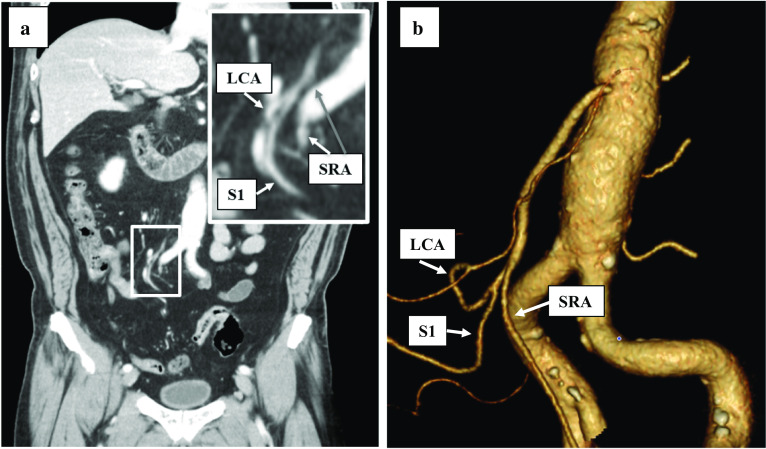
Case 4. a An enhanced CT showed LCA and S1, SRA branched radially from IMA. Enlarged view is shown. b 3D-CT showed LCA and S1, SRA branched radially from IMA
